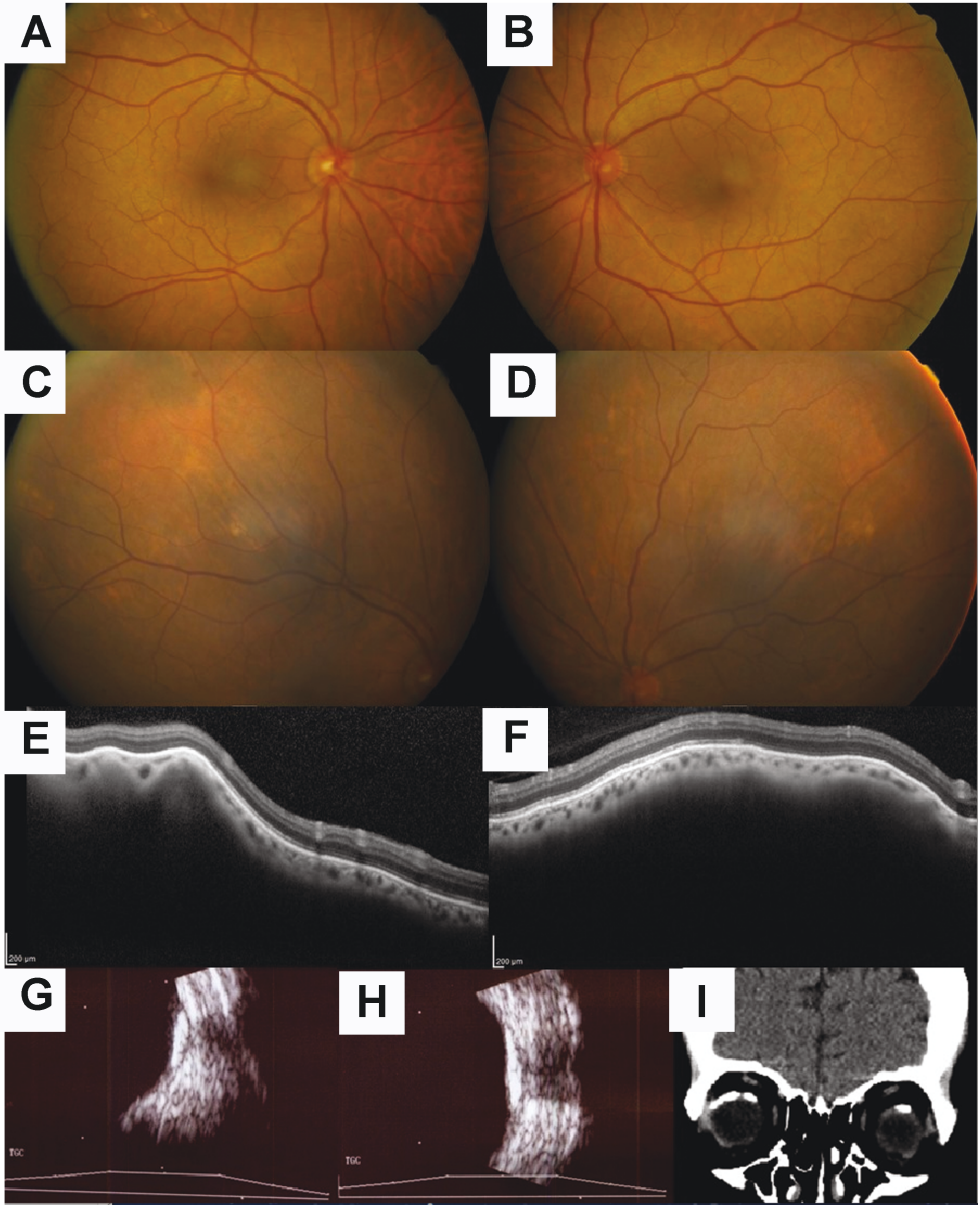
A to D, Ocular examination demonstrating unremarkable posterior poles of the A, right, and B, left, eyes, with yellow deposits superior to the vascular arcades in the A, right, and D, left, eyes. E and F, Optical coherence tomographic scanning through the deposits demonstrates choroidal elevation with overlying normal retina and focal pattern in the E, right eye, and diffuse pattern in the F, left eye. G and H, B-scan ultrasound demonstrates echogenic foci with posterior shadowing in the G, right, and H, left, eyes. I, Coronal computed tomography brain scanning demonstrates sclerochoroidal calcification in both eyes.
